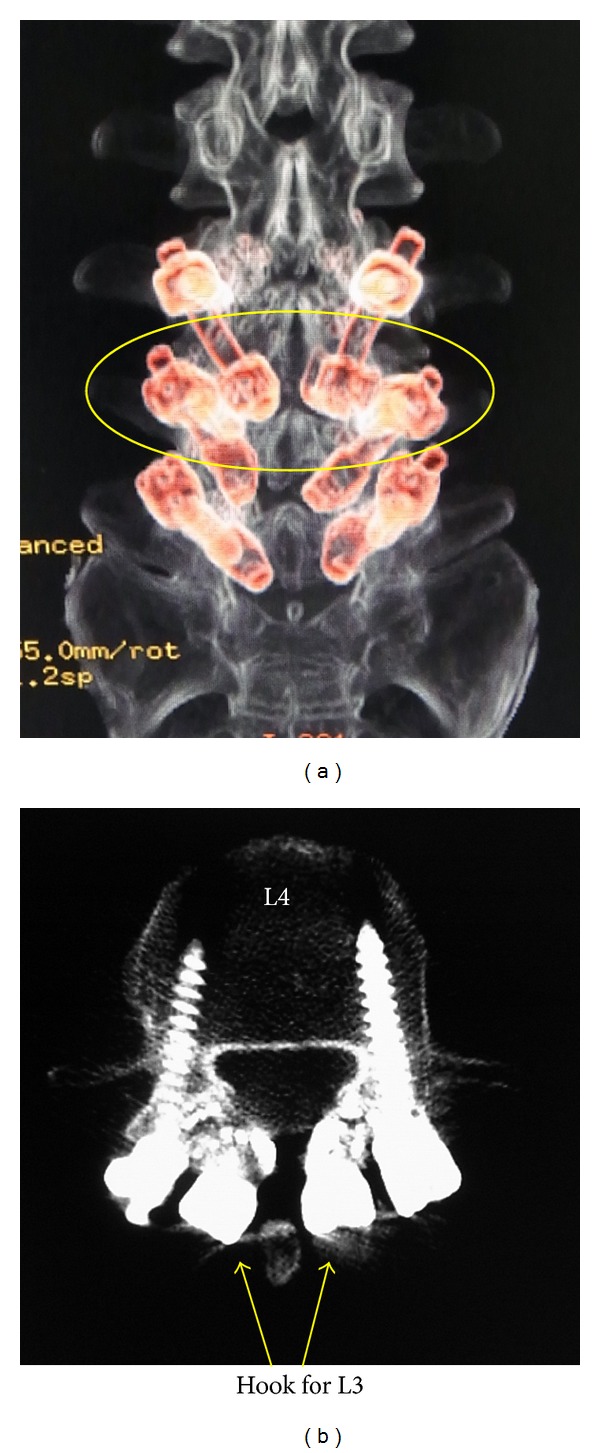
CT after the surgery. Note the proximity of the hook head to L3 and the pedicle screw head to L4.
